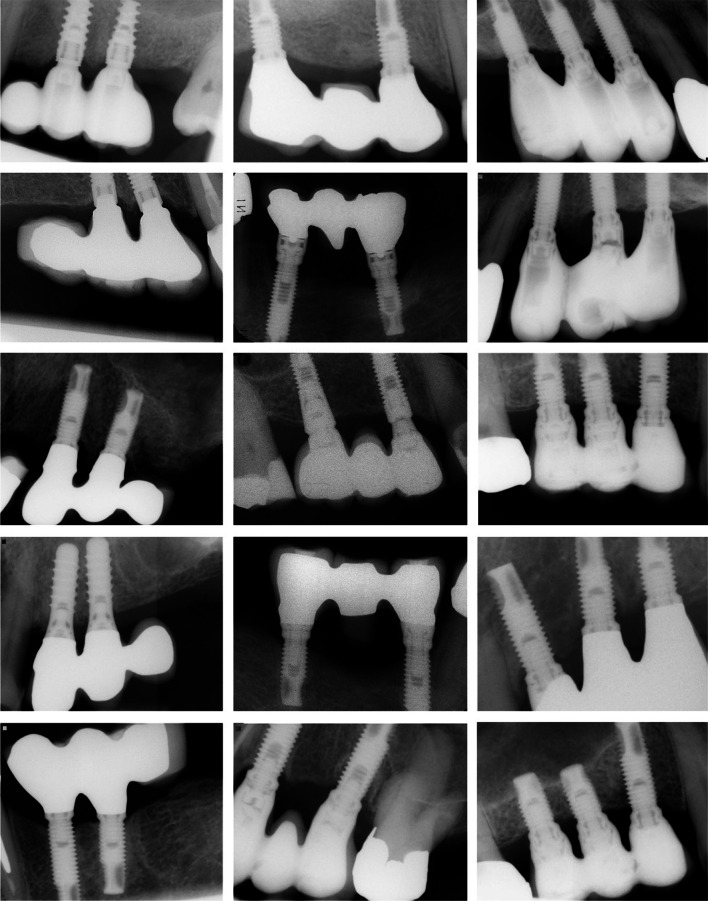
Examples of radiographs of cases included in the present study: 3-unit prosthesis supported by two implants with a cantilever (left column), 3-unit prosthesis supported by two implants with a pontic (middle column), and 3-unit prosthesis supported by three implants (right column)
